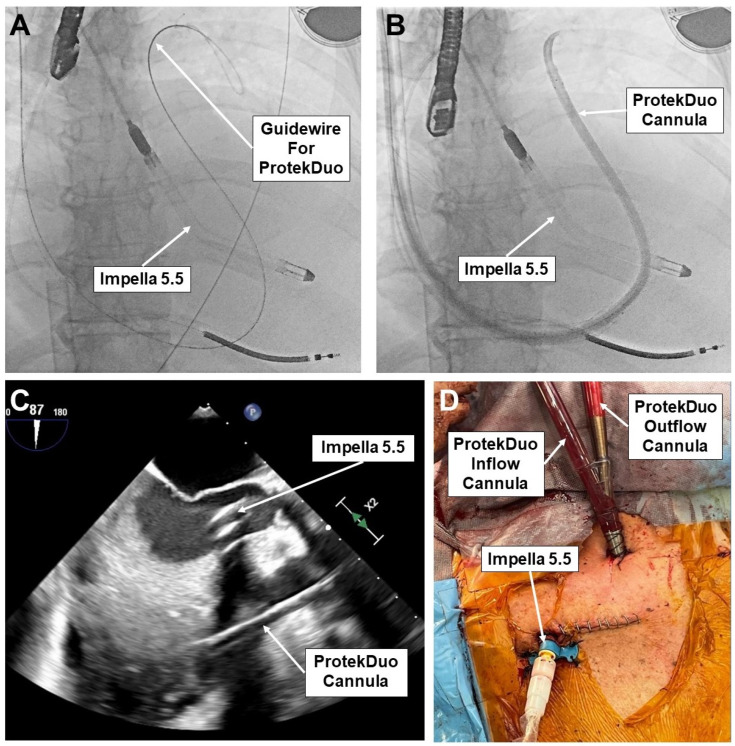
Fluoroscopy image of Impella 5.5 in place and advancing of a guidewire into the pulmonary artery for the ProtekDuo cannula (A). Fluoroscopy image of Impella 5.5 and ProtekDuo cannula (with introducer) in place (B). Transesophageal echocardiographic view of both Impella 5.5 and ProtekDuo cannula in place (C). Intraoperative view after completion of the procedure and instauration of Hybrid BiVAD support (D).
